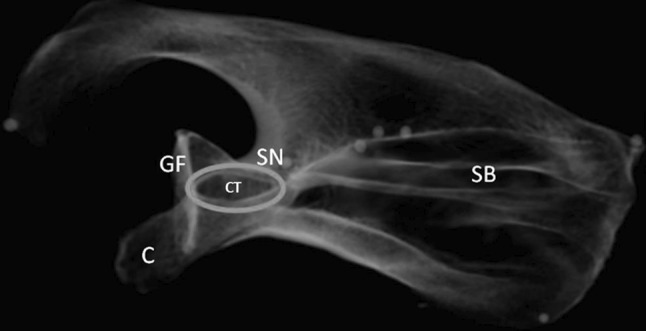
The optimal view of the coracoid tunnel (CT) is based on four anatomical landmarks: coracoid (C), glenoid fossa (GF), scapular notch (SN) and superior scapular border (SB)
